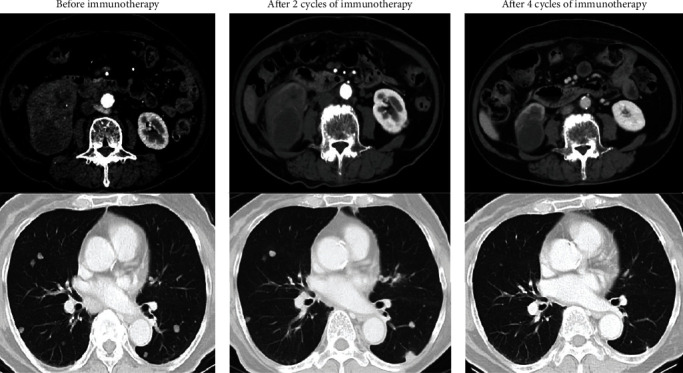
Computed tomography (CT) findings in Case 2 showing new lesions in the lung after two cycles of immunotherapy, which shrank following the two additional cycles.
